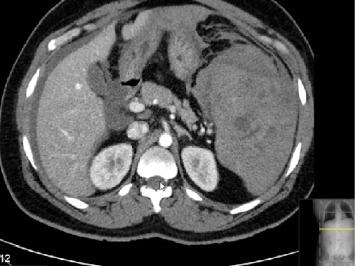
Contrast-enhanced abdominopelvic CT scan showing splenic hematoma with capsular breach.
